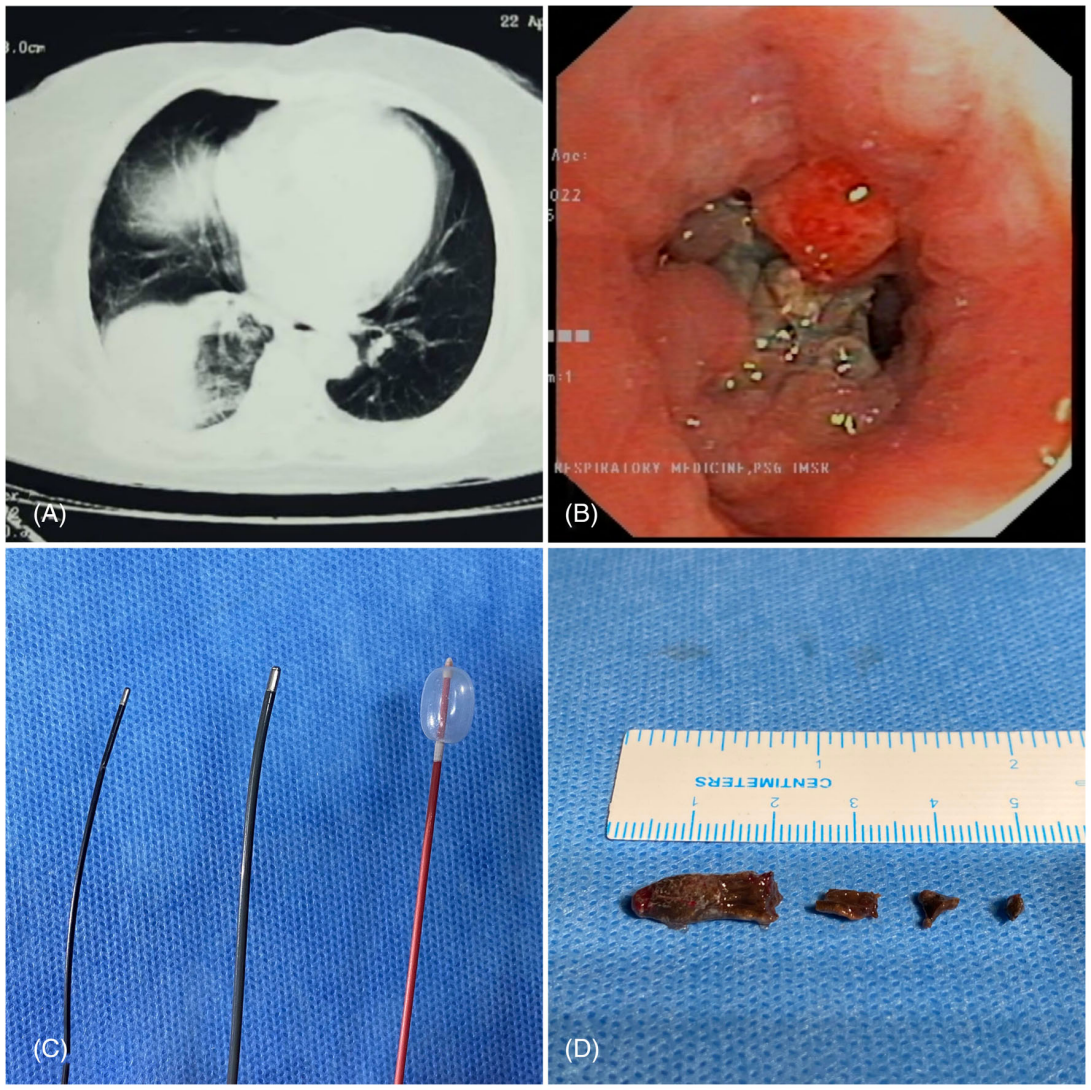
(A) CT chest showing mass like consolidation in the right lower lobe, (B) endobronchial view showing impacted foreign body material with granulation tissue in RB10, (C) 1.1 mm, 1.7 mm Erbe cryoprobe and 4 Fr Fogarty balloon, and (D) retrieved clove
